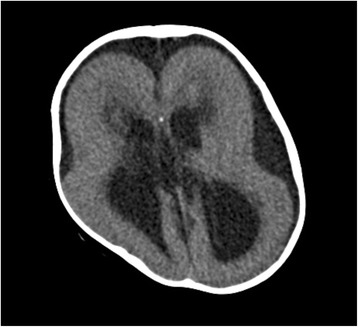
Head computed tomography (CT). Head CT showing type I lissencephaly with midline calcification
